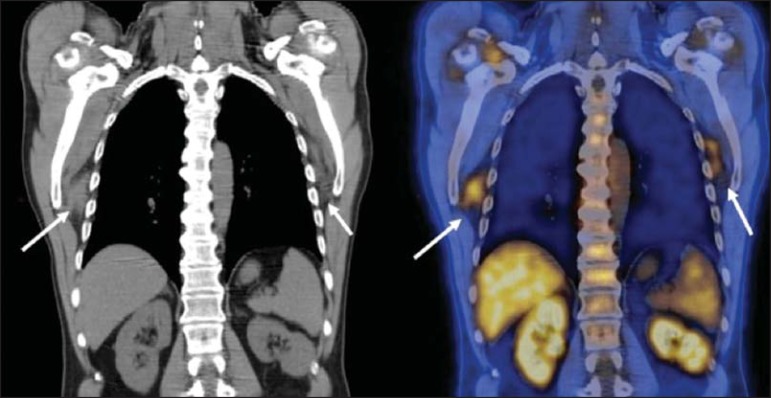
A 78-year-old woman submitted to PET/CT for the staging of a cervical
